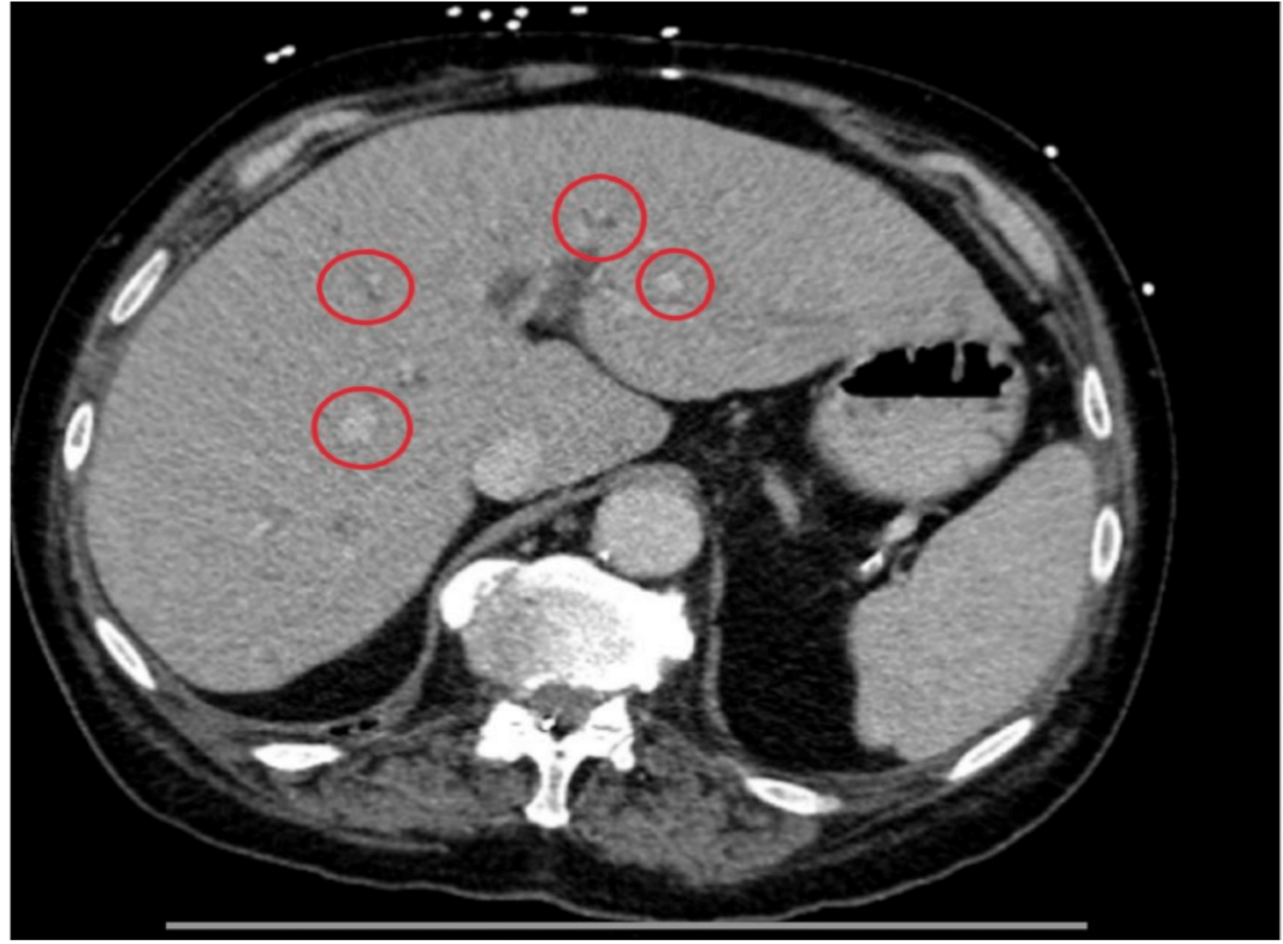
CT of the abdomen and pelvis revealing liver metastatic disease.
